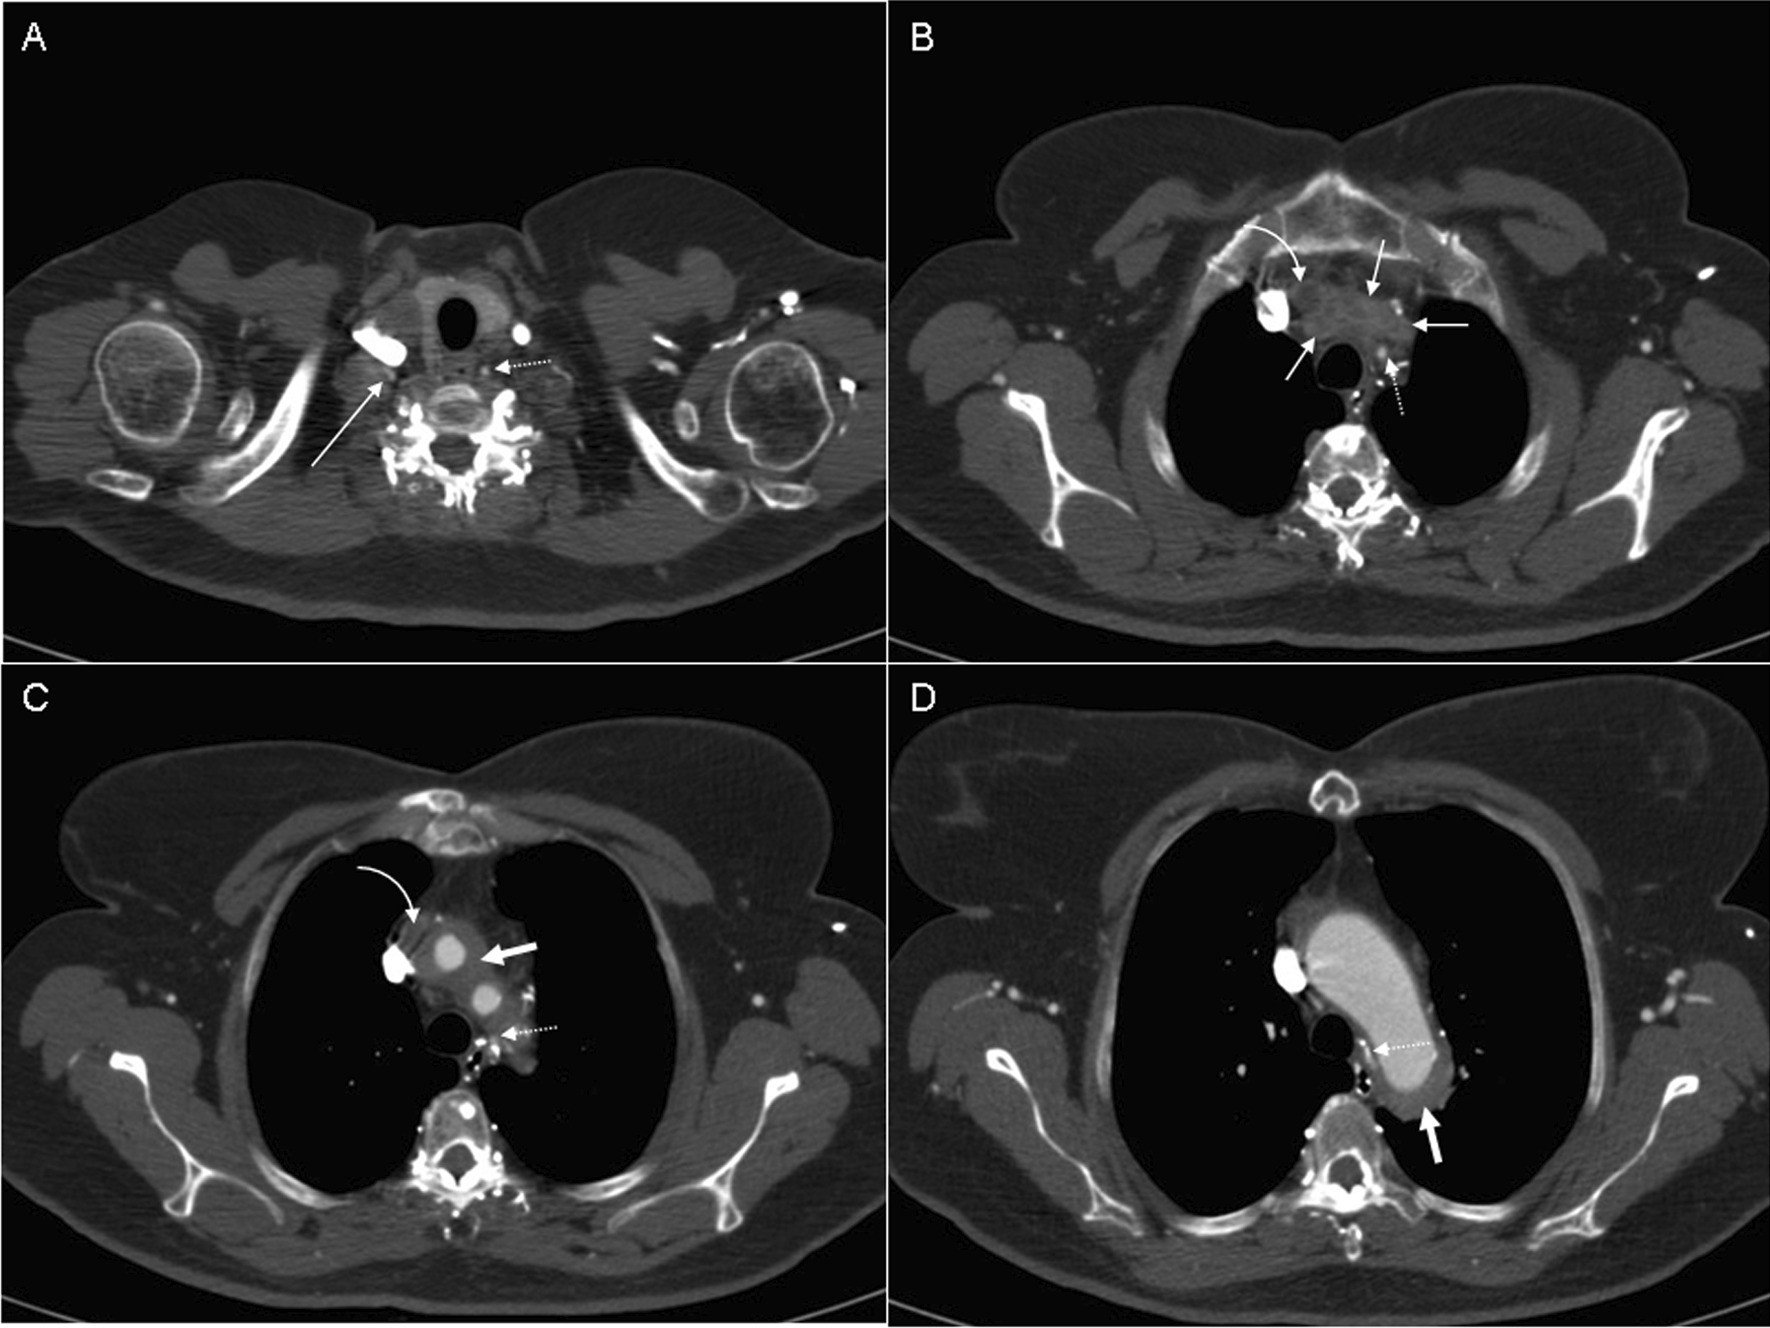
Selected axial CTA images (64 slice CT scanner) in craniocaudal order. Thickened vessel wall and infiltration (thick arrow C, D) surrounding the aortic arch and occluded supra-aortic vessels (thin arrows B) and occluded aorto-carotid bypass (curved arrow B, C). The collateral artery (dotted arrow A–D) arising from the right coronary artery is connected to the left vertebral artery that is one of the few vessels delivering cerebral blood supply. An additional collateral artery arising from the intercostal arteries feeds the right subclavian artery and thereby the right vertebral artery (long thin arrow A). Both subclavian arteries depend on collateral blood supply derived from intercostal collaterals
